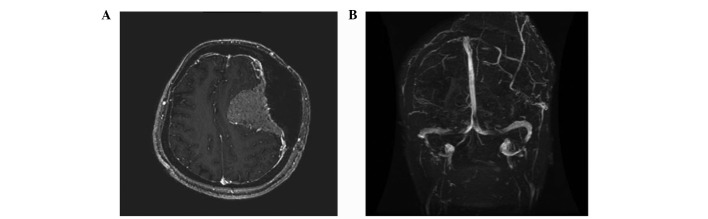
(A) Head magnetic resonance imaging revealed a bilateral frontoparietal bone hyperostosis, particularly in the left side and a cone-shaped intracranial meningioma below the bone hyperostosis. (B) Magnetic resonance angiography showed that blood to was being supplied to the lesions by the external carotid artery and that the superficial temporal artery was abnormally large. In addition, the superficial veins of the left frontoparietal lobes had been compressed downward.
